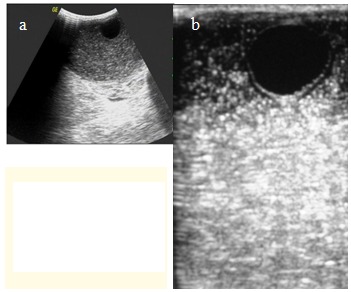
Ultrasonography revealed daughter cysts in the right thigh
